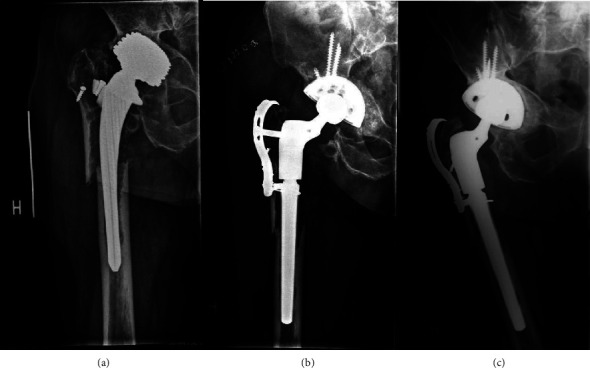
Radiographs showing (a) preoperative anterior to posterior view of the right femur with aseptic loosening and fracture of an uncemented femoral component and Paprosky type IIIB bone loss; (b) rTHA using an Arcos tapered, modular femoral component with a with a trochanter reattachment plate; (c) 2 years follow-up showing a well fixed osseointegrated Arcos component with restoration of proximal femoral bone stock.
